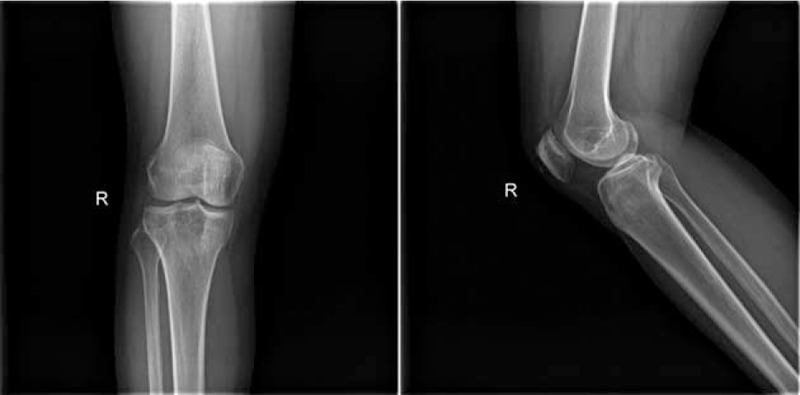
Plain radiograph of the right knee reveals a sharply defined isolated lytic lesion with sclerotic borders. No cortical destruction, periosteal reaction, or soft-tissue component are present.
